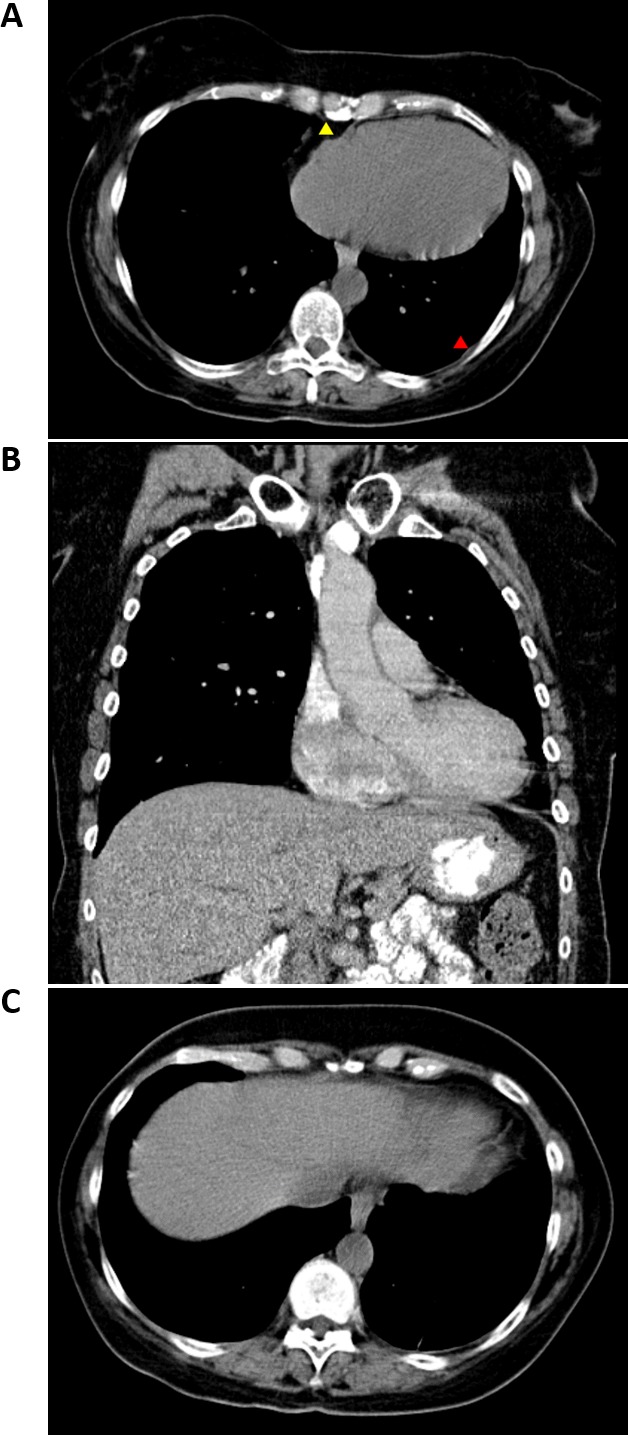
CT imaging of cardiac angiosarcoma subsequent to propranolol treatment (A) Axial chest CT following treatment with non-selective beta blocker shows marked reduction in size of the pericardial and pleural mass. There is minimal residual pleural (red arrowhead) and pericardial thickening (yellow arrowhead). (B) Coronal chest CT shows regression of soft tissue mass encasing the ascending aorta and main pulmonary artery after treatment with non-selective beta blocker. (C) Axial CT abdomen following treatment with non-selective beta blocker shows resolution of liver metastases.
